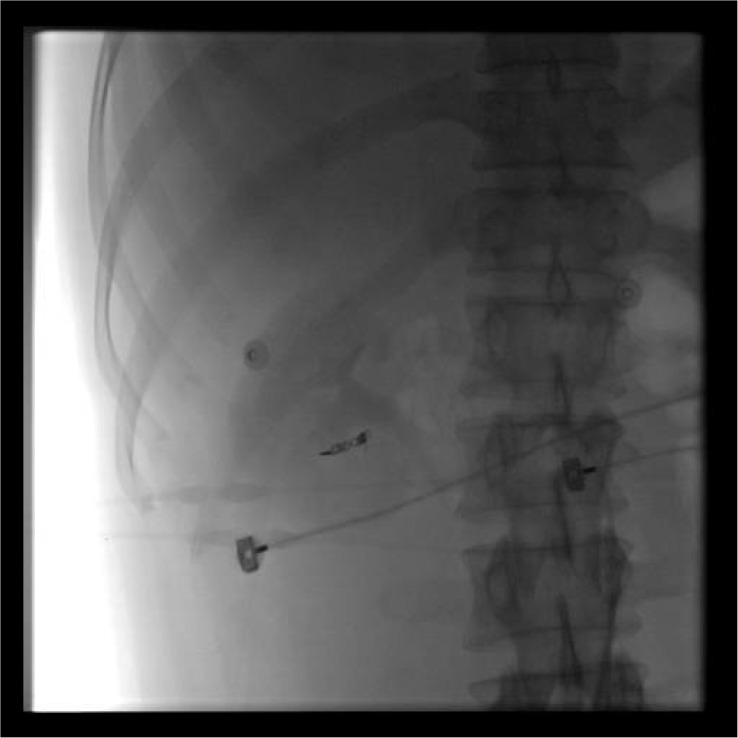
Post-interventional treatment of the right renal arteriovenous fistula on abdominal plain radiograph.
